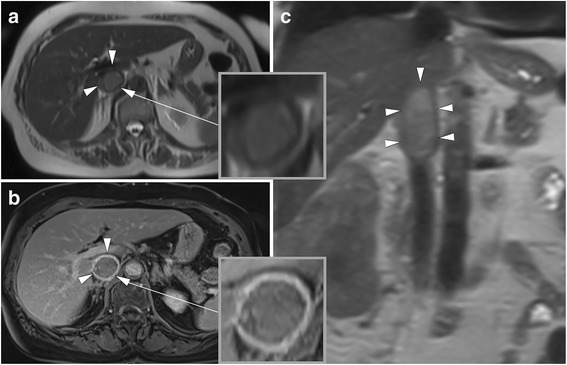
Images in a 79-year old woman with a clear cell renal cell carcinoma (RCC) and bland inferior vena cava (IVC) thrombus without wall invasion. The RCC extends from the right kidney into the infrahepatic IVC. a axial T2-weighted HASTE image. b T1-weighted contrast-enhanced 3D GRE (VIBE) image and (c), the coronal T2-weighted HASTE image for anatomic reference. Note that the thrombus is floating in the IVC and that there is no complete obstruction of the caval lumen (a, c). The contrast-enhanced image (b) demonstrates that there is no enhancement of the tumor thrombus, contact to or breach of the vessel wall, so that IVC wall invasion appears unlikely. During extended nephrectomy, this thrombus could be easily removed from the IVC without necessitating segmental resection. HASTE = Half-Fourier-acquired singe-shot turbo spin echo, VIBE = Volumetric interpolated breath-hold examination
